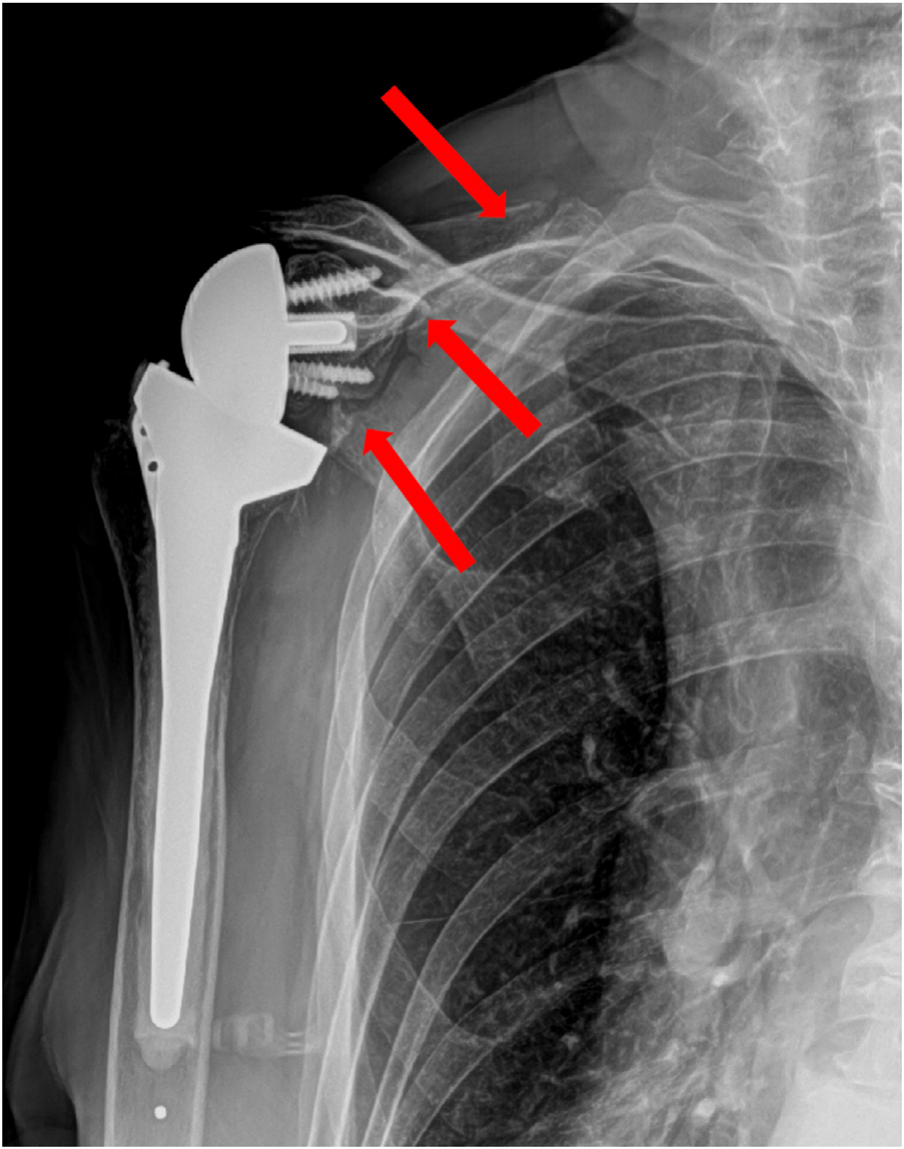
X-ray Grashey view demonstrating a scapular fracture from the inferior scapular neck and exiting the medial scapular spine.
